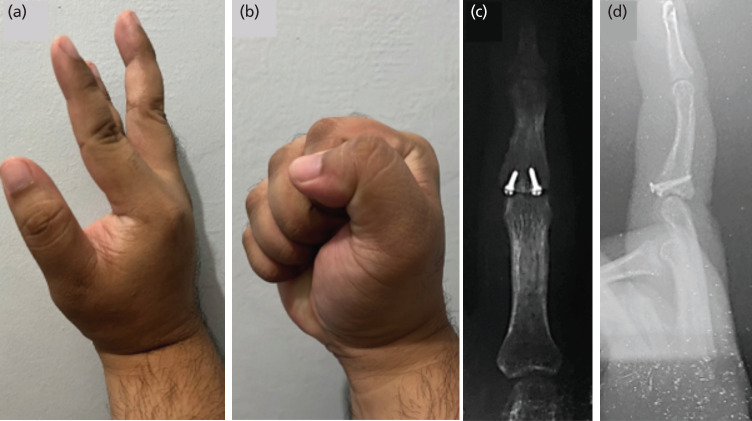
(a) 10-month post-operative outcome of finger range of motion demonstrating full PIPJ extension, and (b) flexion up to 90° and (c, d) post-operative radiographs showing bony union and concentric PIPJ joint reduction.
